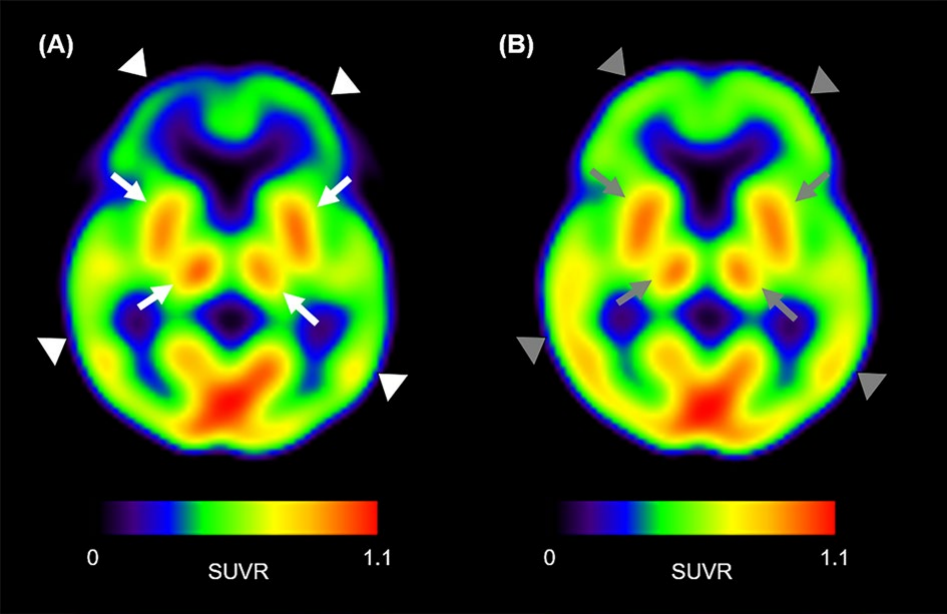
Representative images of early-phase PETs. The ^18^F-FP-CIT SUVR image showed less uptake in cortical areas (white arrowheads in A) than the ^18^F-flutemetamol SUVR image (grey arrowheads in B), while the central structures showed similar activity in the two PETs (white arrows in A and grey arrows in B).
